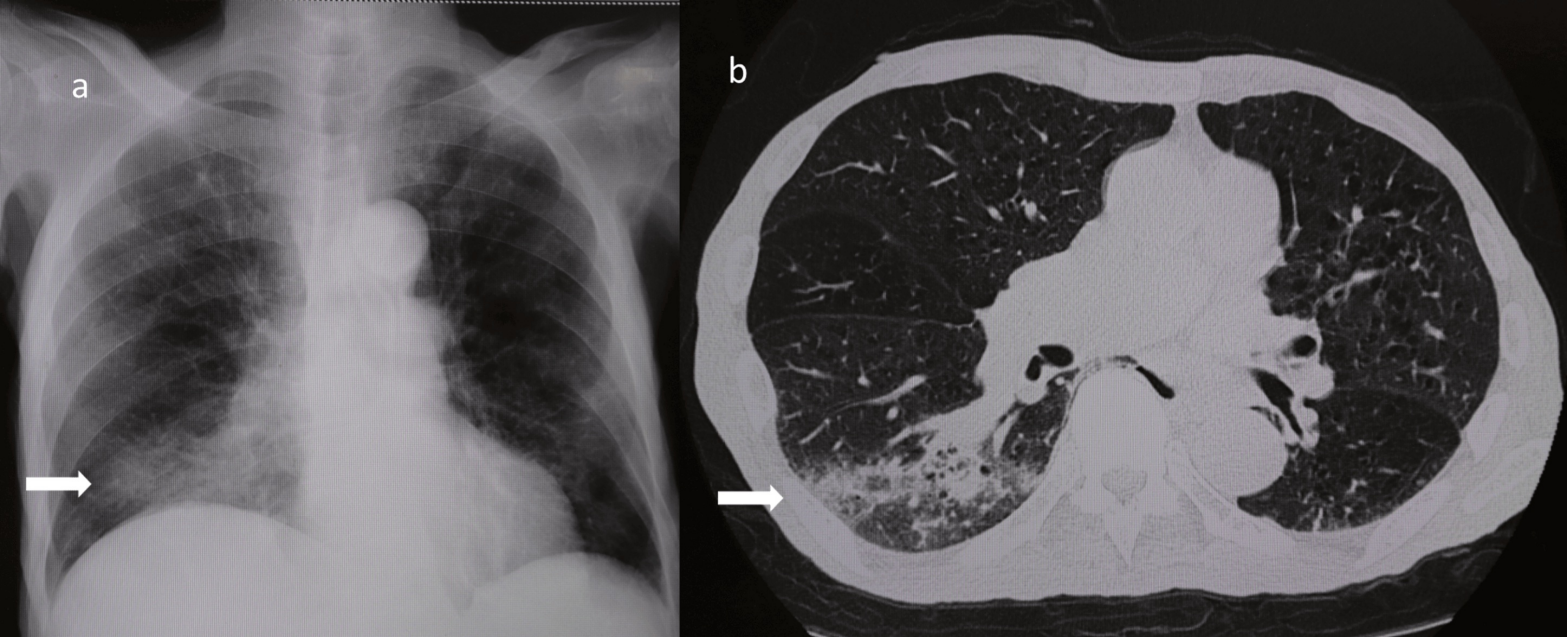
Chest radiographic findings a: Chest X-ray on admission showing right-sided pulmonary infiltration. b: Chest CT scan demonstrating right-sided pulmonary infiltration. CT: computed tomography
